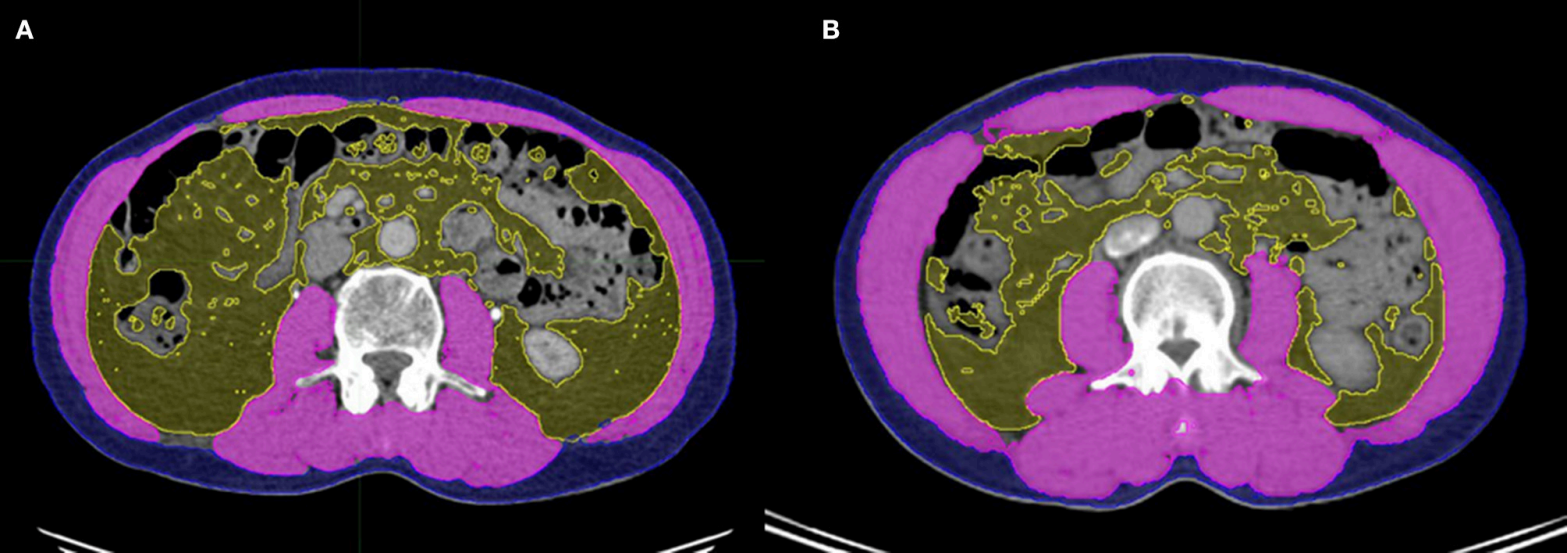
Slices of computed tomography (CT) images of patients with (A) and without (B) sarcopenia who had similar body mass indices. Skeletal muscle, visceral adipose tissue, and subcutaneous adipose tissue were measured using the MIM Vista software (MIM corp., version 6.1, OH, USA).
